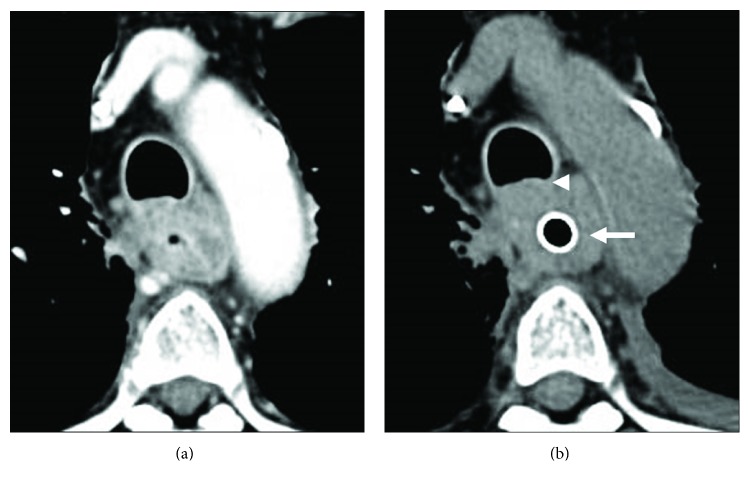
(a) CT findings before stent placement. Two months after chemoradiotherapy, the tumor located in the middle thoracic esophagus had invaded the trachea. (b) CT findings after Niti-S ultrathin stent placement (arrow). The membranous portion of the trachea did not transform (arrowhead).
